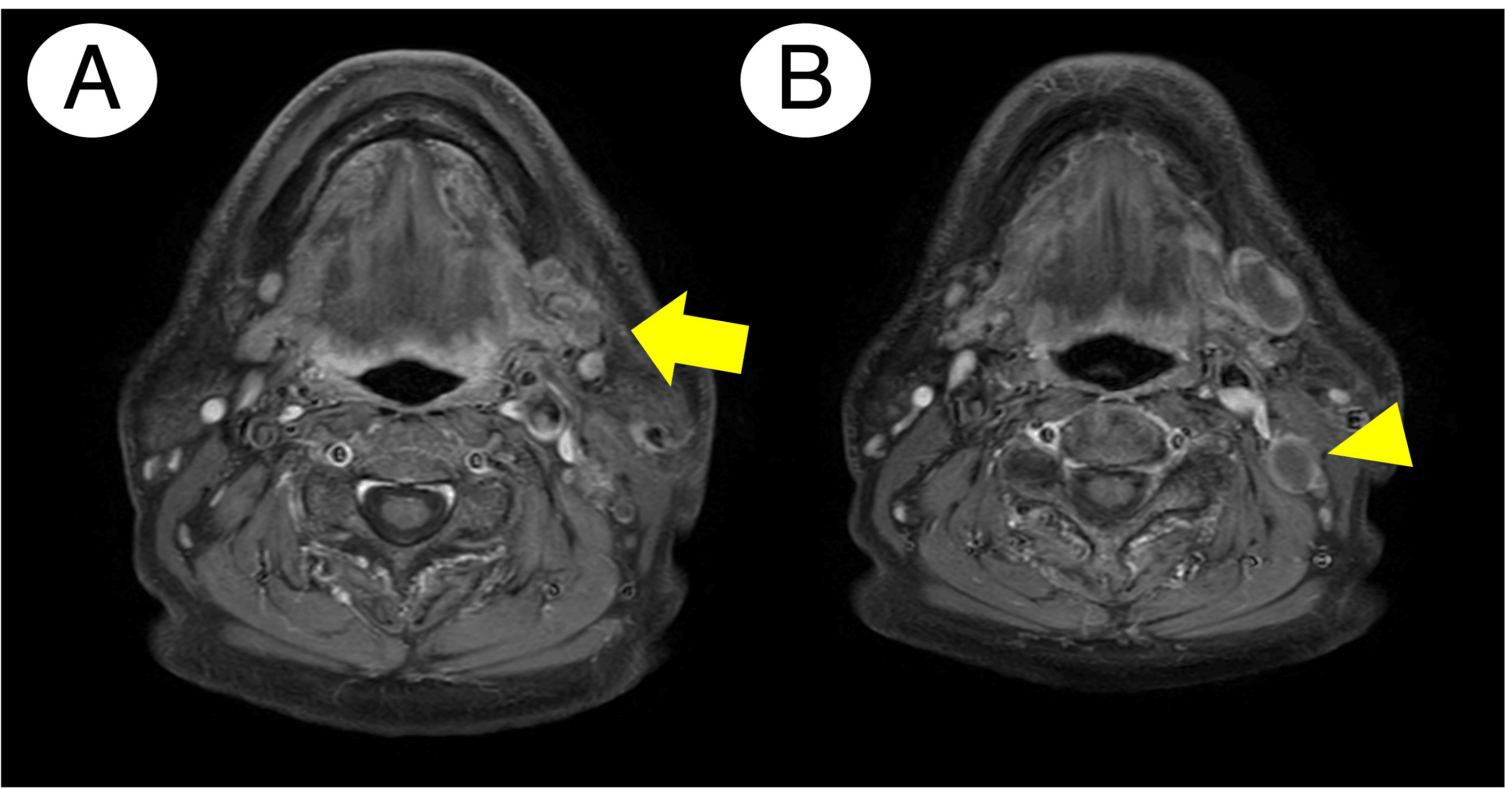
Contrast-enhanced MRI findings at the first appearance A: T1-weighted image with gadolinium contrast revealed that a heterogeneous, markedly enhancing mass lesion infiltrated into the left mandibular marrow (arrow); B: Cervical lymph node displayed rim-enhancement findings, indicating lymph node metastasis (arrowhead shows a representative metastatic lymph node)
